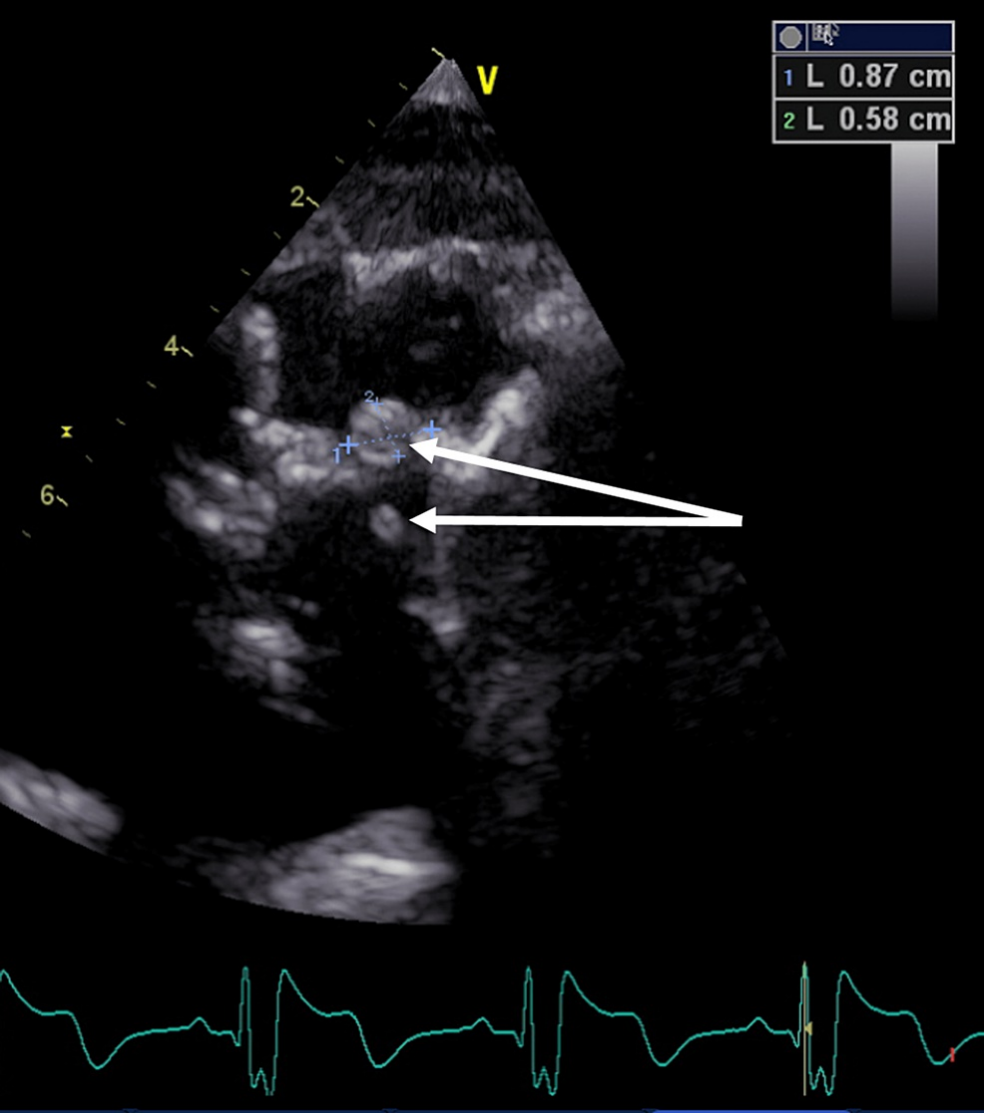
Transthoracic echocardiogram/short-axis view at the basal level White arrows showing pulmonic valve vegetations
